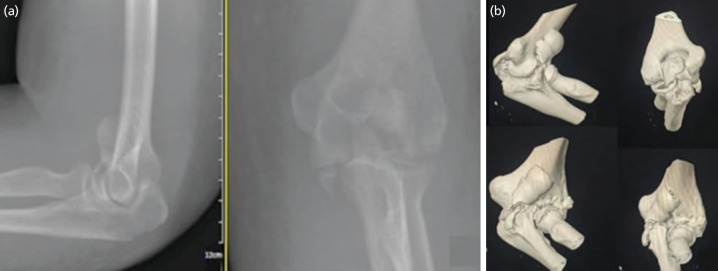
(a) AP and lateral radiograph of left elbow shows comminuted fracture of distal humerus. (b) Computed tomography of left elbow shows the trochlear fragment displaced anteriorly with ipsilateral olecranon fracture.
